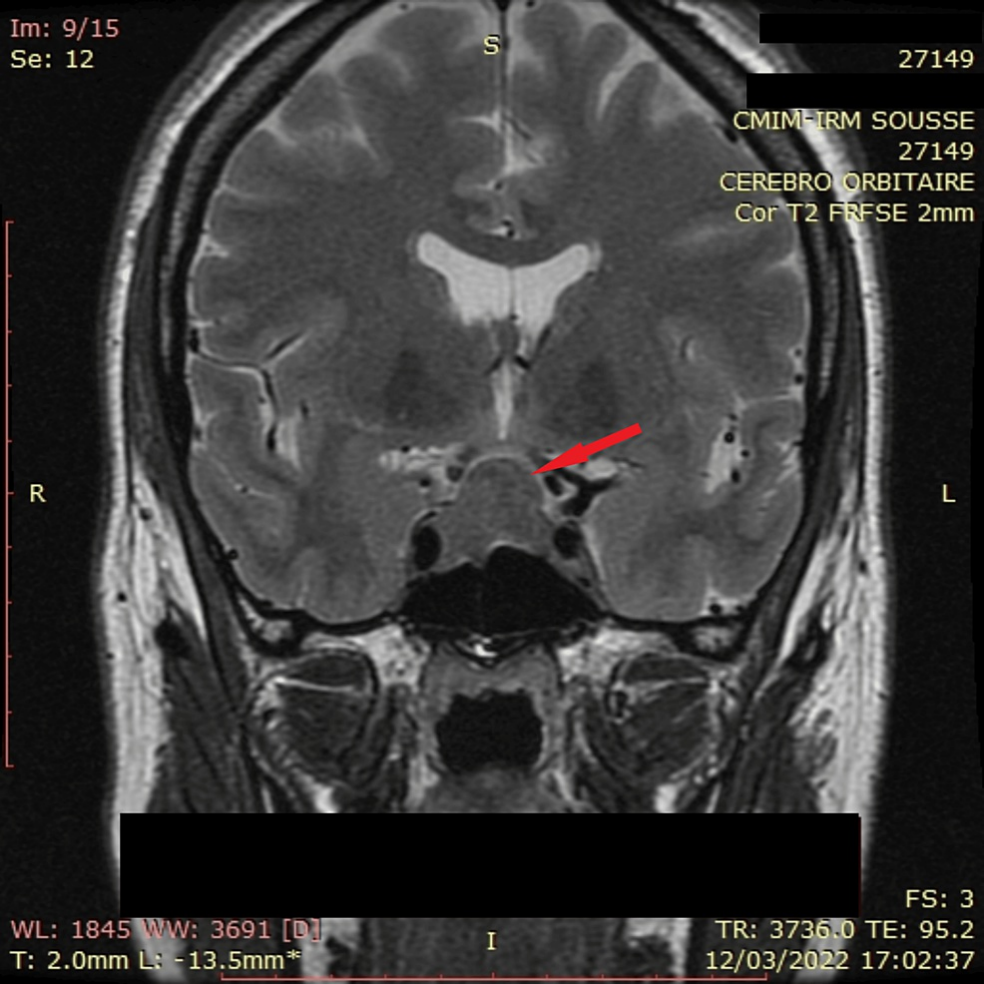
Preoperative hypothalamic-pituitary MRI coronal section T2-weighted coronal section of pituitary adenoma showing suprasellar extension with compression of the optic chiasm and inferior extension in contact with the right cavernous sinus, which includes the carotid artery (Knosp 2) as indicated by the red arrow.
